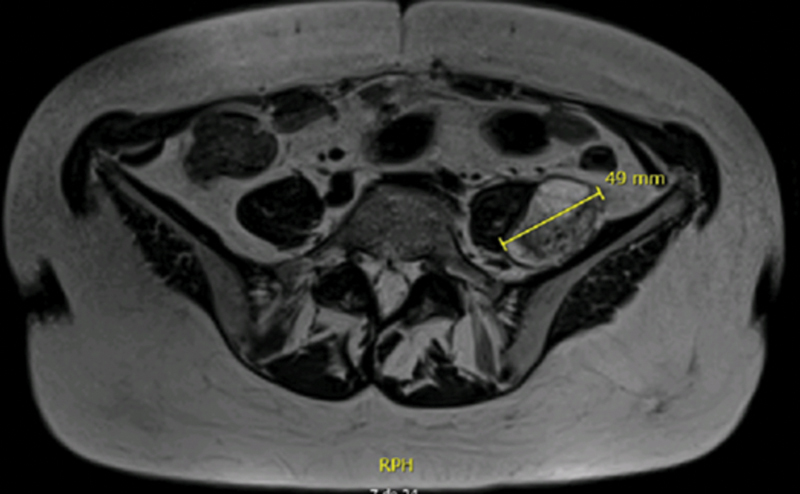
MRI (axial plane) – T2- weighted sequence: heterogeneously hyperintense lesion, with few cystic areas, suggestive of neurogenic lumbar plexus tumour.
